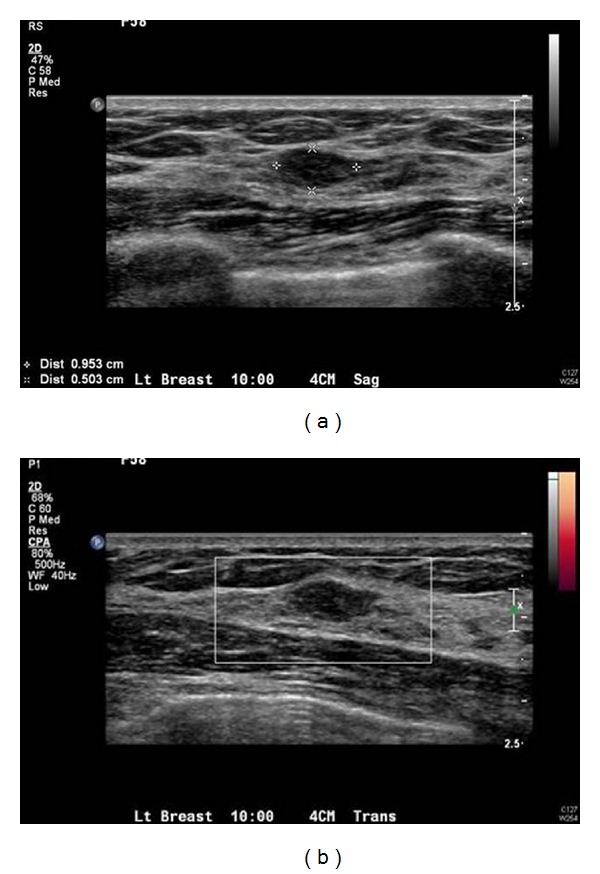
(a) Ultrasound evaluation in the upper inner breast demonstrates an oval, hypoechoic lesion, corresponding to the lesion seen on MRI (though slightly larger in dimension) with minimally irregular margins. (b) No increased vascularity.
